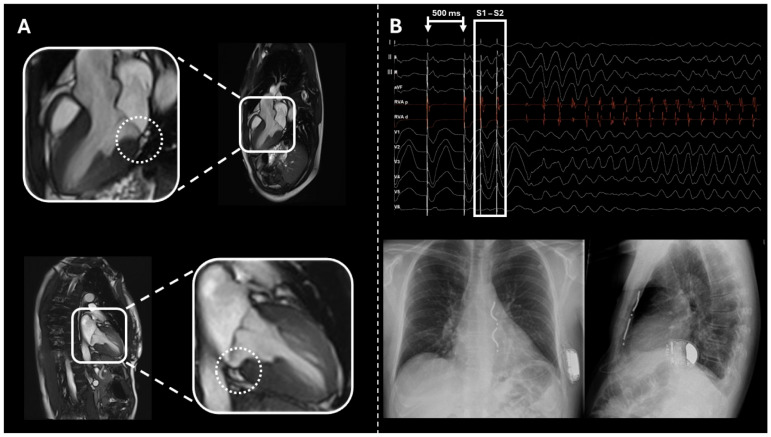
(A) Cardiac magnetic resonance imaging in the three-chamber view (upper panel) and two-chamber view (lower panel) demonstrating a mitral annular disjunction (MAD) measuring 6 mm, evident as a separation between the posterior mitral annulus and the basal inferolateral left ventricular myocardium during systole. (B) Upper section: Intracardiac electrophysiological study performed using a drive train of 8 stimuli at a cycle length of 500 ms, followed by 2 ventricular extrastimuli (S1–S2) at a coupling interval of 220 ms, resulting in the induction of ventricular fibrillation (VF). The arrhythmia was promptly terminated with a single 200 J external biphasic defibrillation shock, restoring sinus rhythm. Lower section: Anteroposterior and lateral chest radiographic views following implantation of an extravascular implantable cardioverter–defibrillator (EV-ICD), demonstrating appropriate lead and device placement.
